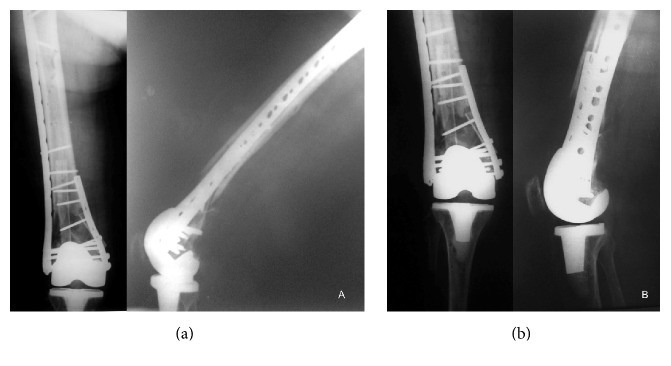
Immediate postoperative X-rays showing good reduction of the fracture, anatomic alignment of the articular surface, and rigid fixation. (a) Note the nonvascularized fibular strut graft. The larger piece was used to bridge the metaphyseal defect and the small piece to replace the medial wall of the distal femur. (b) The alignment of the knee joint was anatomically restored.
